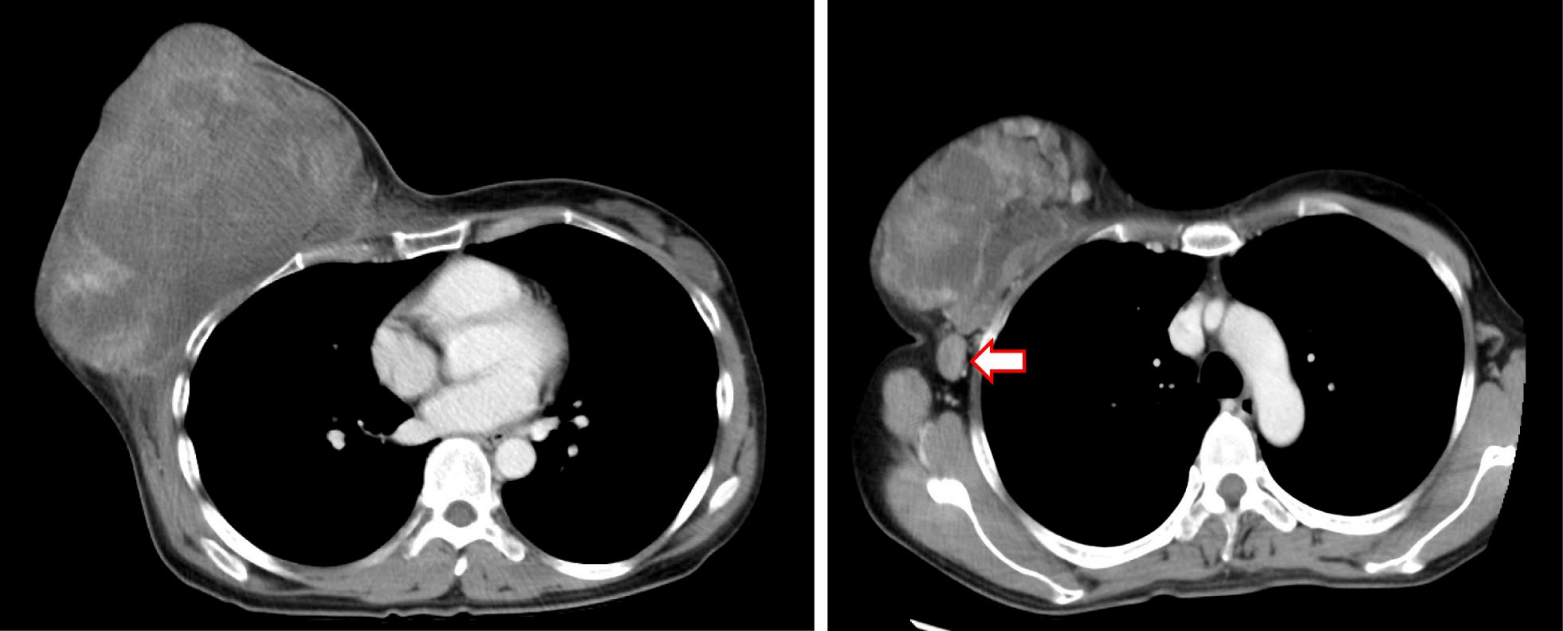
Computed tomography showed a 20 cm heterogeneous mass in the right breast and axillary and supraclavicular lymph nodes swelling (arrow)
